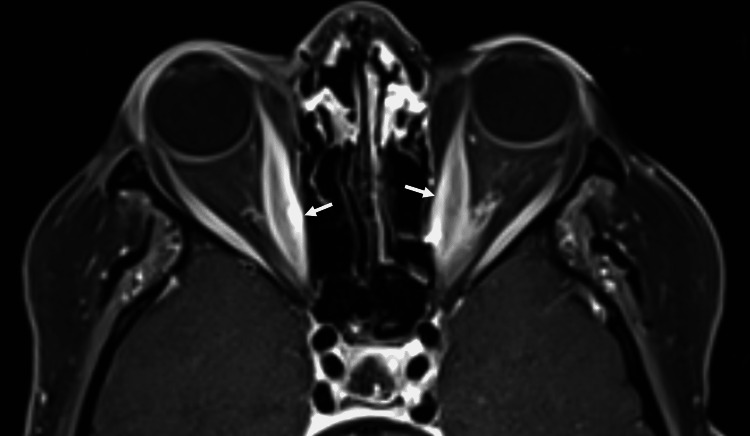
Contrast-enhanced magnetic resonance imaging (MRI) of the orbits showing bilateral ocular proptosis with medial recti muscle enlargement (white arrows).
